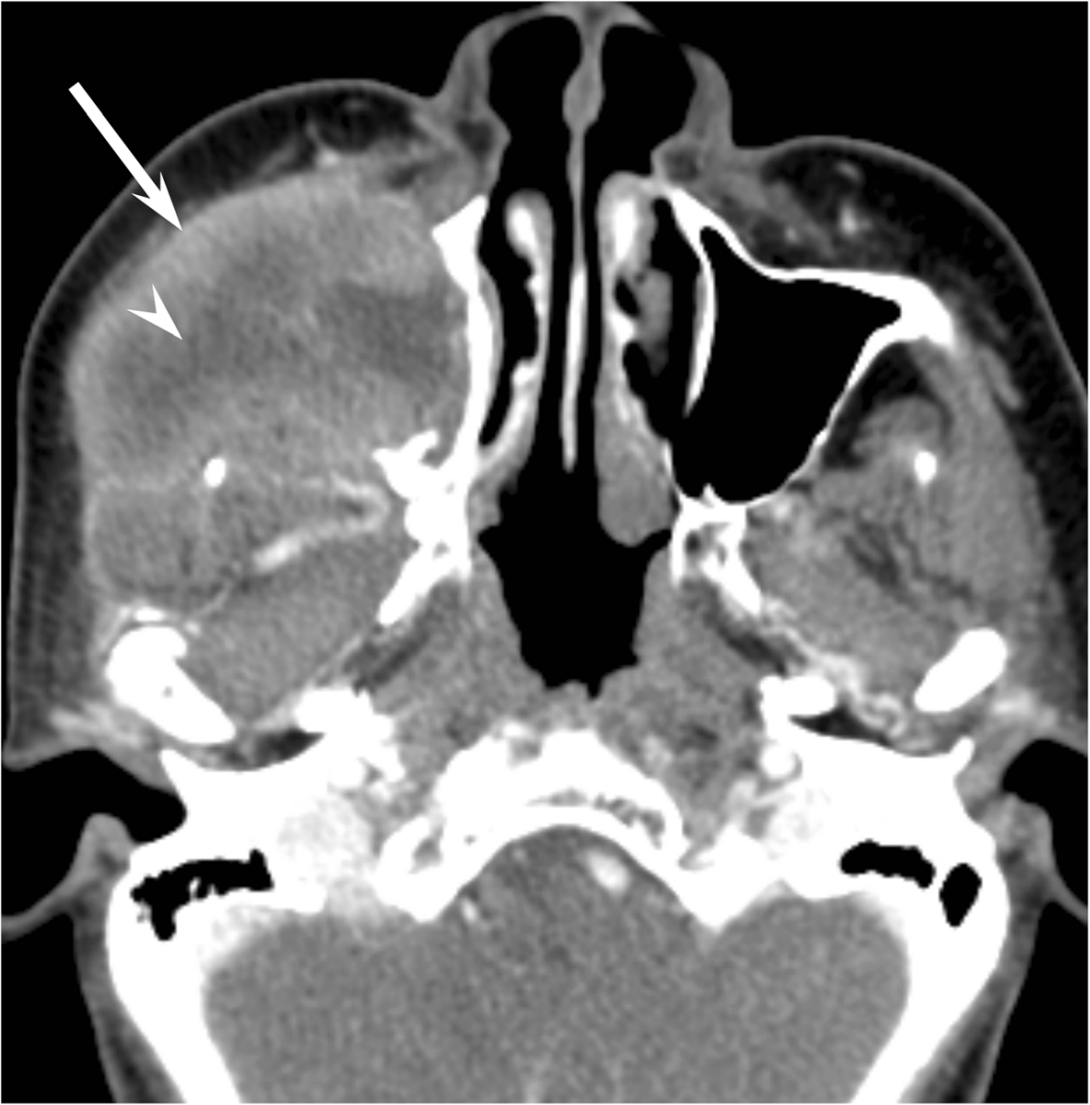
A 60–year-old woman with maxillary sinus squamous cell carcinoma (destructive-type). Enhanced CT image shows heterogeneously enhanced bulky mass (arrow) with unenhanced area suggestive of necrosis (arrowhead). The sinus walls extensively disappear due to the destructive growth
